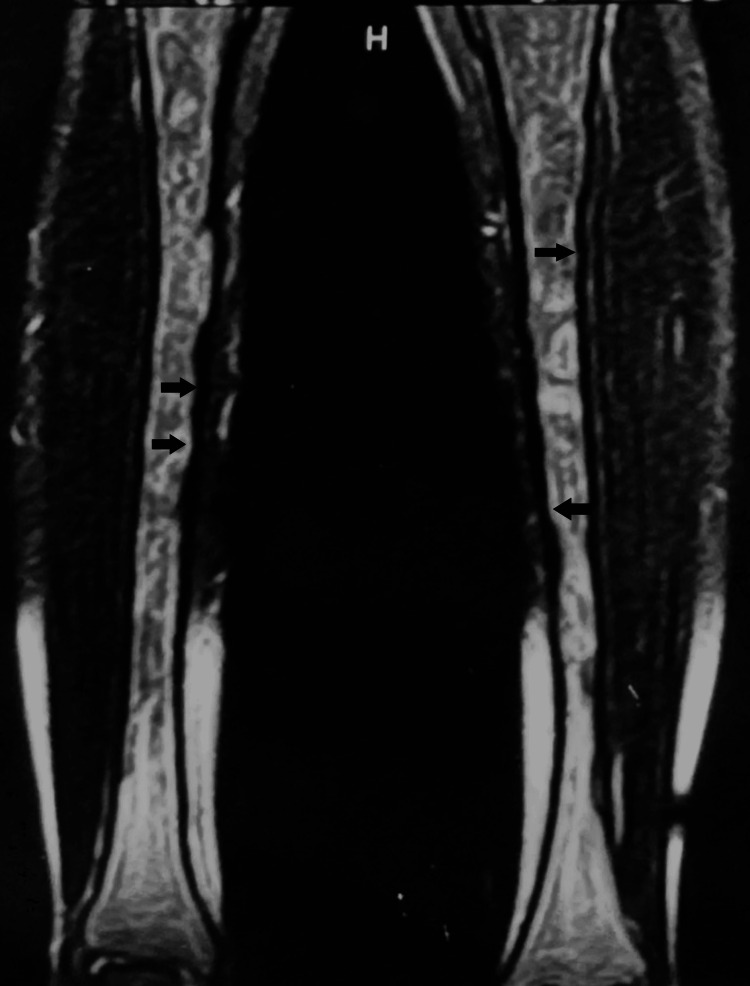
Coronal T2-weighted MR image shows diffuse, markedly heterogeneous signal intensity in tibial diaphysis on both sides. Note endosteal scalloping (arrows) without cortical disruption.
